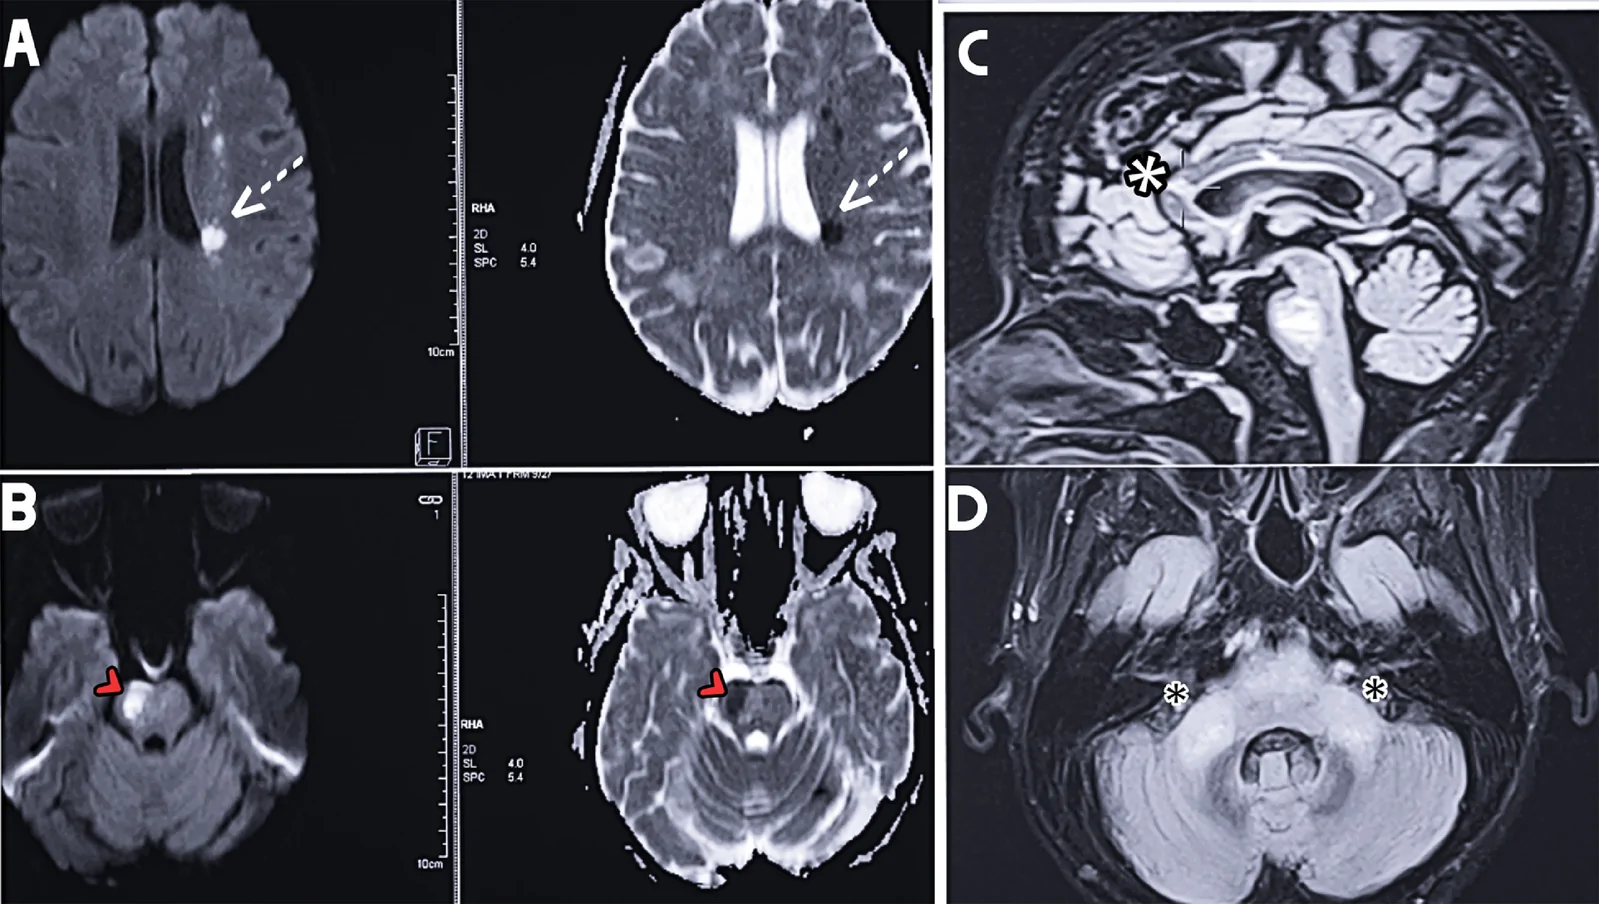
Brain MRI findings. (A) Axial diffusion-weighted image (DWI, left panel) with corresponding apparent diffusion coefficient (ADC) map (right panel) at the level of the corona radiata. The white dashed arrow indicates a focal area of high signal on DWI with corresponding low ADC signal, consistent with diffusion restriction and acute ischemic infarction in the left corona radiata, within the territory of the left middle cerebral artery. (B) Axial DWI (left panel) with corresponding ADC map (right panel) at the level of the pons. The red arrowhead indicates a focal area of diffusion restriction in the right paramedian pons, consistent with a second acute ischemic infarct in the posterior circulation territory, confirming simultaneous multiterritory infarction. (C) Sagittal T2-weighted image at the midline. The asterisk (*) indicates a focal T2 hyperintensity in the genu of the corpus callosum consistent with chronic ischemic gliosis. Note the markedly reduced caliber of the brainstem tegmentum at the level of the pons, reflecting severe atrophic changes in the context of chronic vertebrobasilar occlusive disease. (D) Axial T2-weighted image at the level of the middle cerebellar peduncles (MCPs). Bilateral asterisks () indicate symmetric, confluent T2 hyperintensities involving both MCPs, consistent with chronic ischemic gliosis secondary to severe vertebrobasilar occlusive disease, rather than acute infarction.
